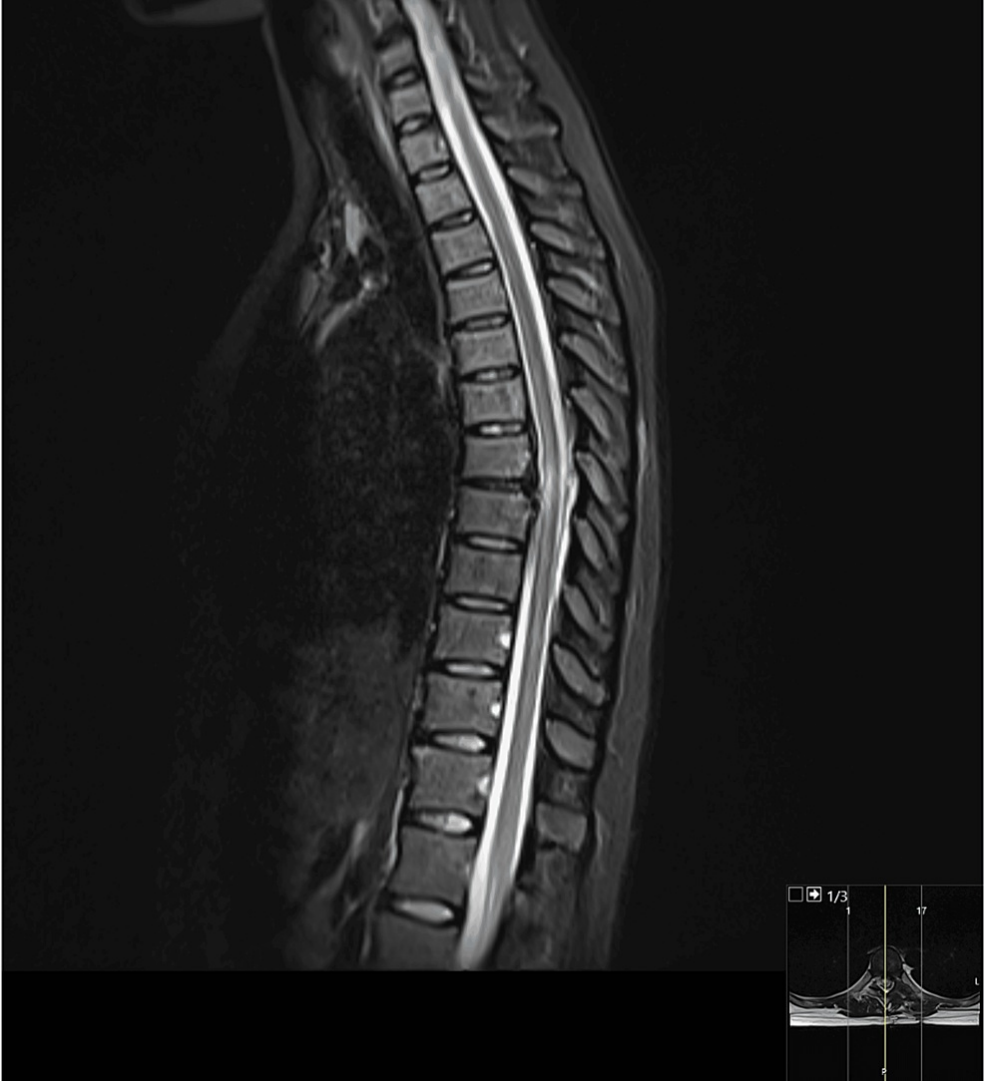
MRI two months after surgery, with no signs of recurrence.
